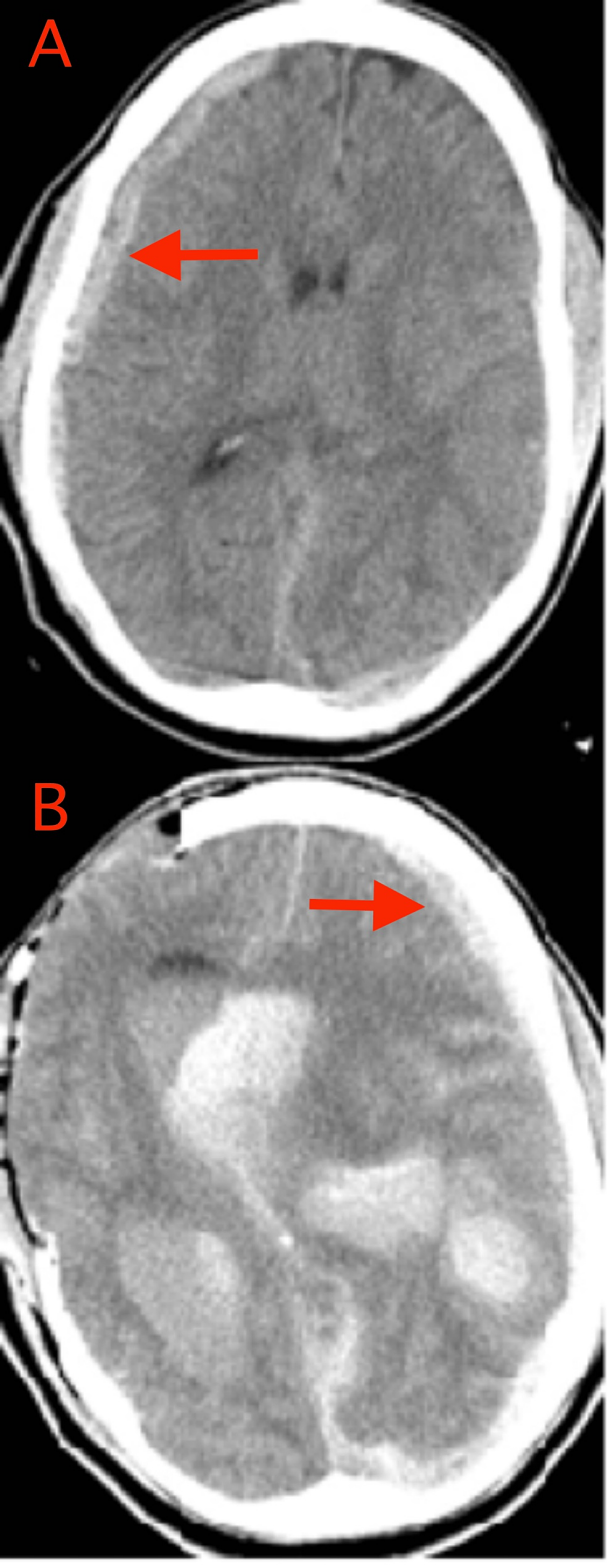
CT images demonstrating DTICH type 2 CT images for a patient experiencing DTICH type 2 are shown. The top image (panel A) represents the pre-operative CT scan, which demonstrated a right-sided subdural hematoma (red arrow in panel A). The patient underwent a right hemicraniectomy and evacuation of the right-sided subdural hematoma, and the bottom image (panel B) represents the postoperative CT scan performed immediately following surgery. The bottom image (panel B) shows a new left-sided intracerebral hematoma, intraventricular hemorrhage, and left-sided subdural hematoma (red arrow in panel B). CT: computerized tomography; DTICH: delayed traumatic intracerebral hematoma
